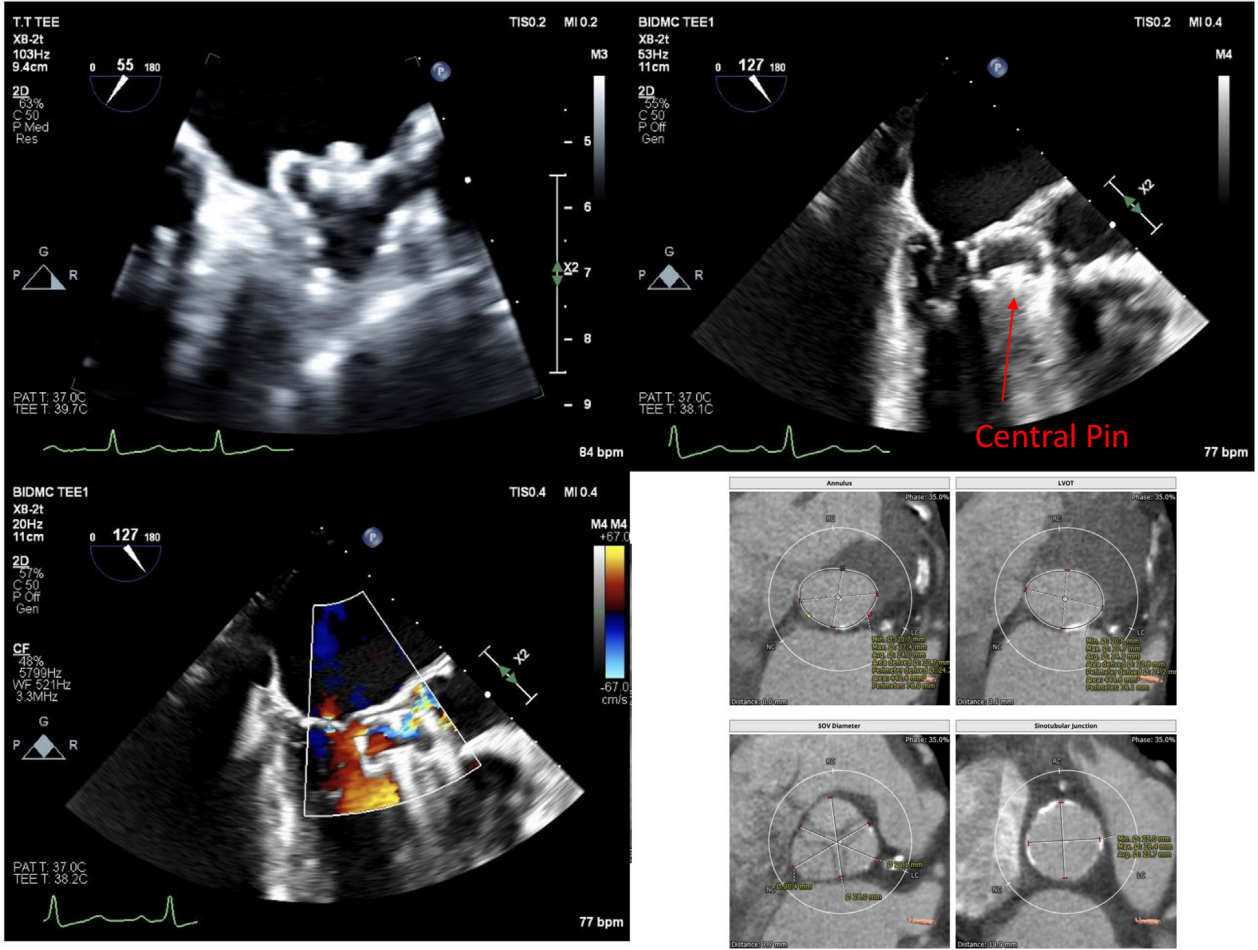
Pre-Intervention Imaging (Top left) Outside hospital implantation transesophageal echocardiogram (TEE) at 45° demonstrating a well-seated 24-mm Watchman FLX device within the left atrium in the left atrial appendage with postcompression measurements of ∼20 mm (17% compression). (Top right) Intraoperative extraction TEE at 130° demonstrating migrated Watchman device with central pin (arrow) toward the ventricular septum in the left ventricular outflow tract (LVOT) with (bottom left) color Doppler showing aortic regurgitation with flow through the Watchman and trivial mitral regurgitation. (Bottom right) 3Mensio (Pie Medical Imaging) report of the aortic valve measuring the annulus, LVOT, sinus, and sinotubular junction. LC = left coronary cusp; NC = noncoronary cusp; RC = left coronary cusp.
